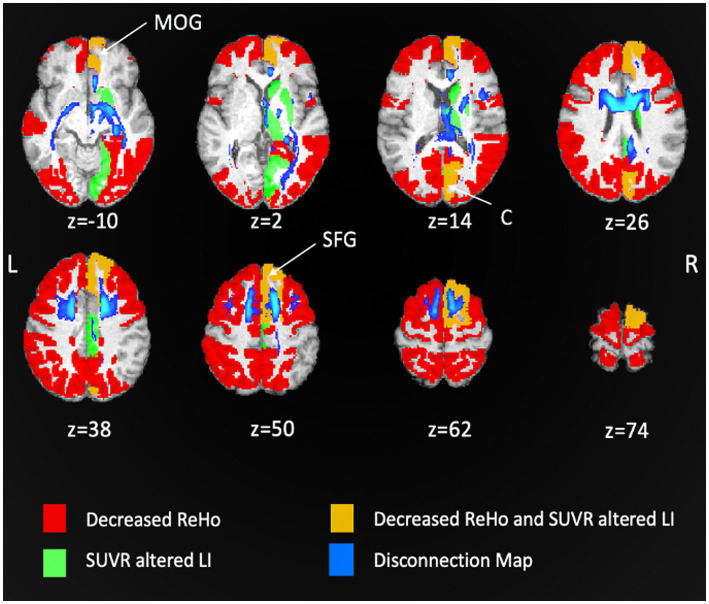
Overlap maps of decreased ReHo, [^18^F]FDG SUVR altered laterality index (LI) and disconnection map. Patient’s regions with decreased ReHo are shown in red, SUVR altered LI in light green. The overlap between decreased ReHo and SUVR altered LI is depicted in orange and corresponds to right medial prefrontal and right medial occipital cortices. The structural disconnection map is shown in blue (MOG, medial orbital gyrus; C, cuneus; SFG, superior frontal gyrus).
